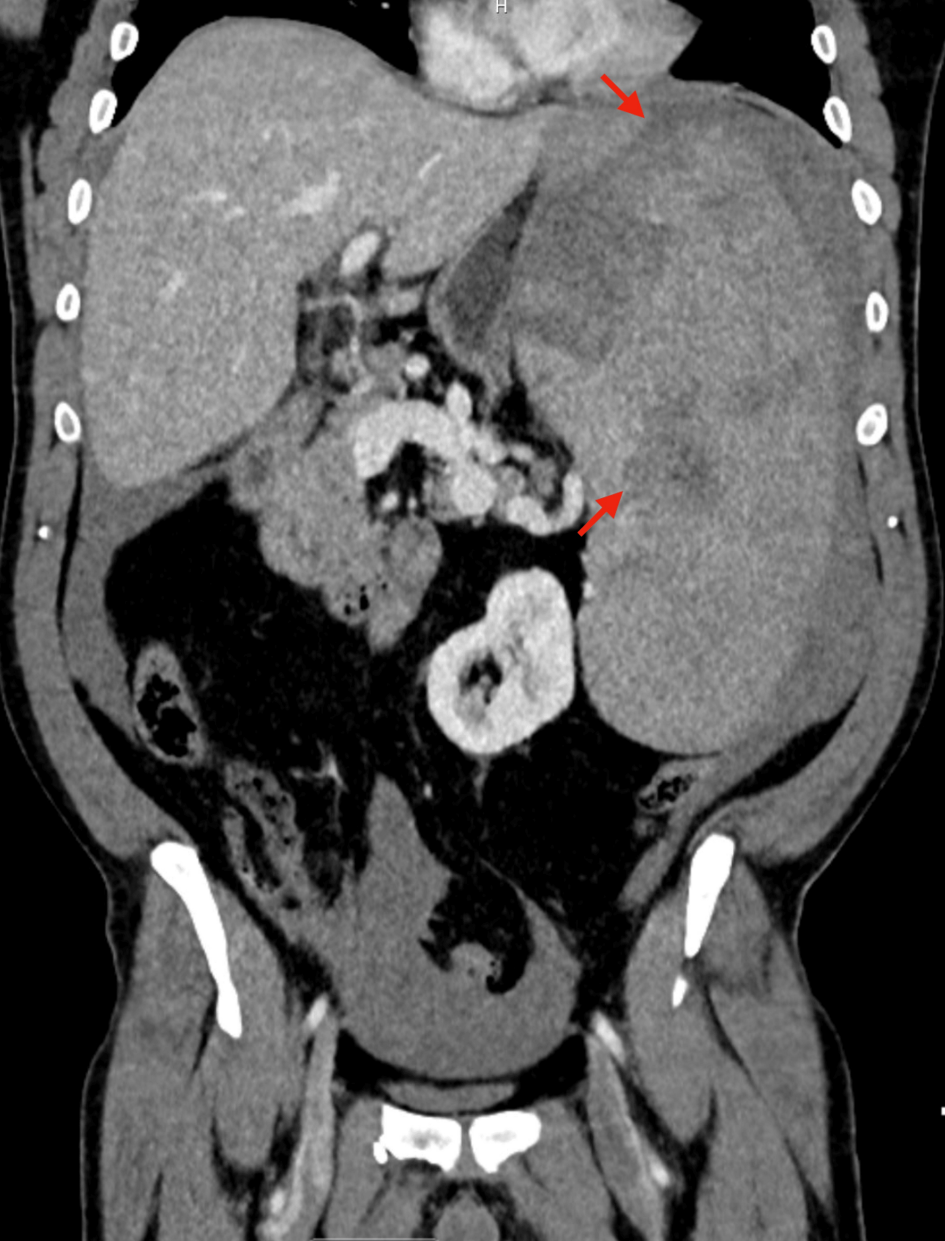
Coronal contrast-enhanced CT demonstrating atraumatic splenic rupture Coronal contrast-enhanced CT of the abdomen showing marked splenomegaly with multifocal parenchymal lacerations (red arrows) and large-volume hemoperitoneum. CT: computed tomography
